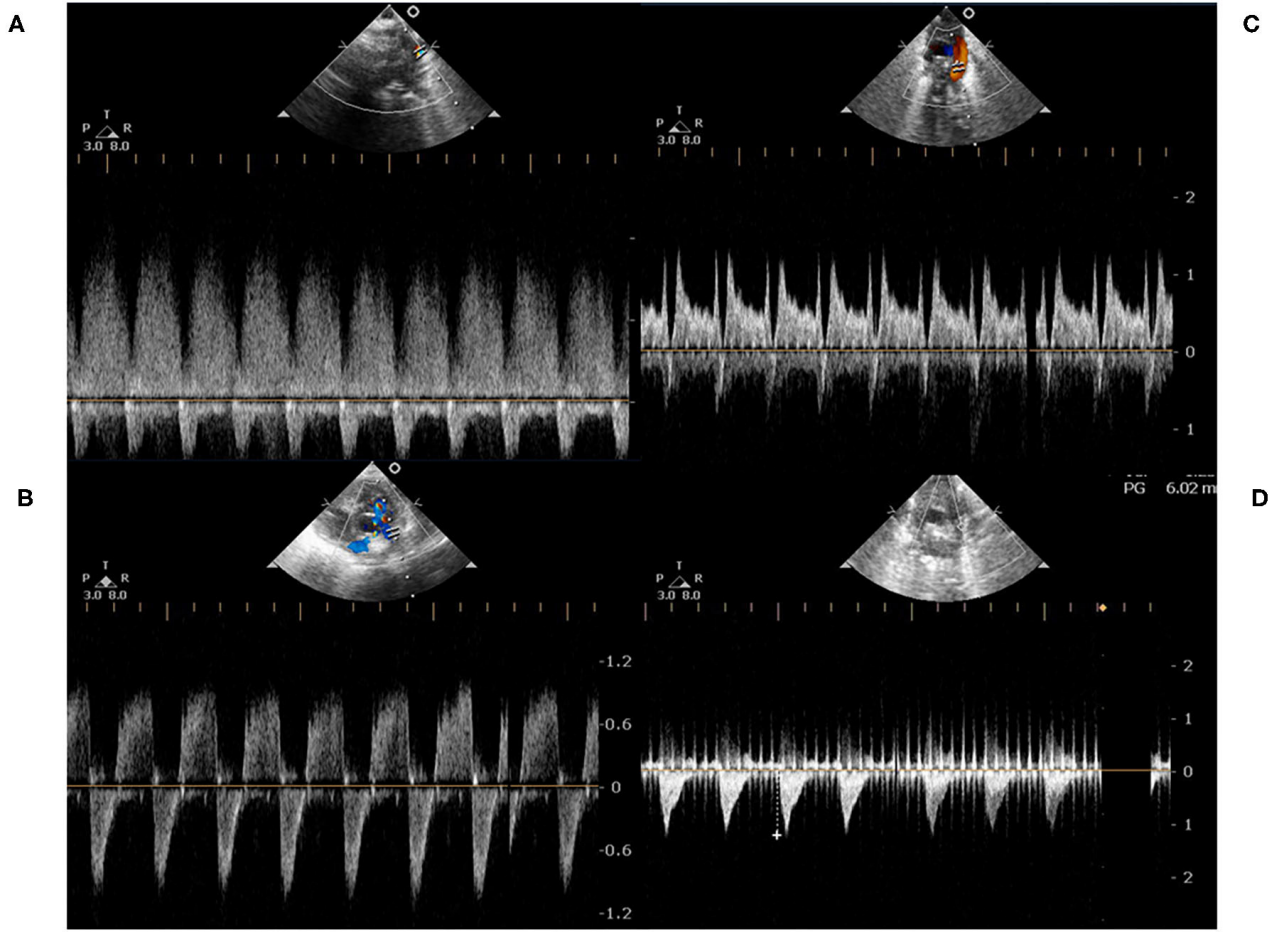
(A) represented a continuous left-to-right shunt of a PDA, which was detected by pulse-wave Doppler. At the end-diastolic phase, there was a persistent left-to-right shunt; (B,C) represented a bidirectional shunt of a PDA. At the end-diastolic phase, the shunting directions were transient right-to-left; (D) represented a right-to-left shunt. There was rare left-to-right shunt either at the systolic or diastolic phase.
